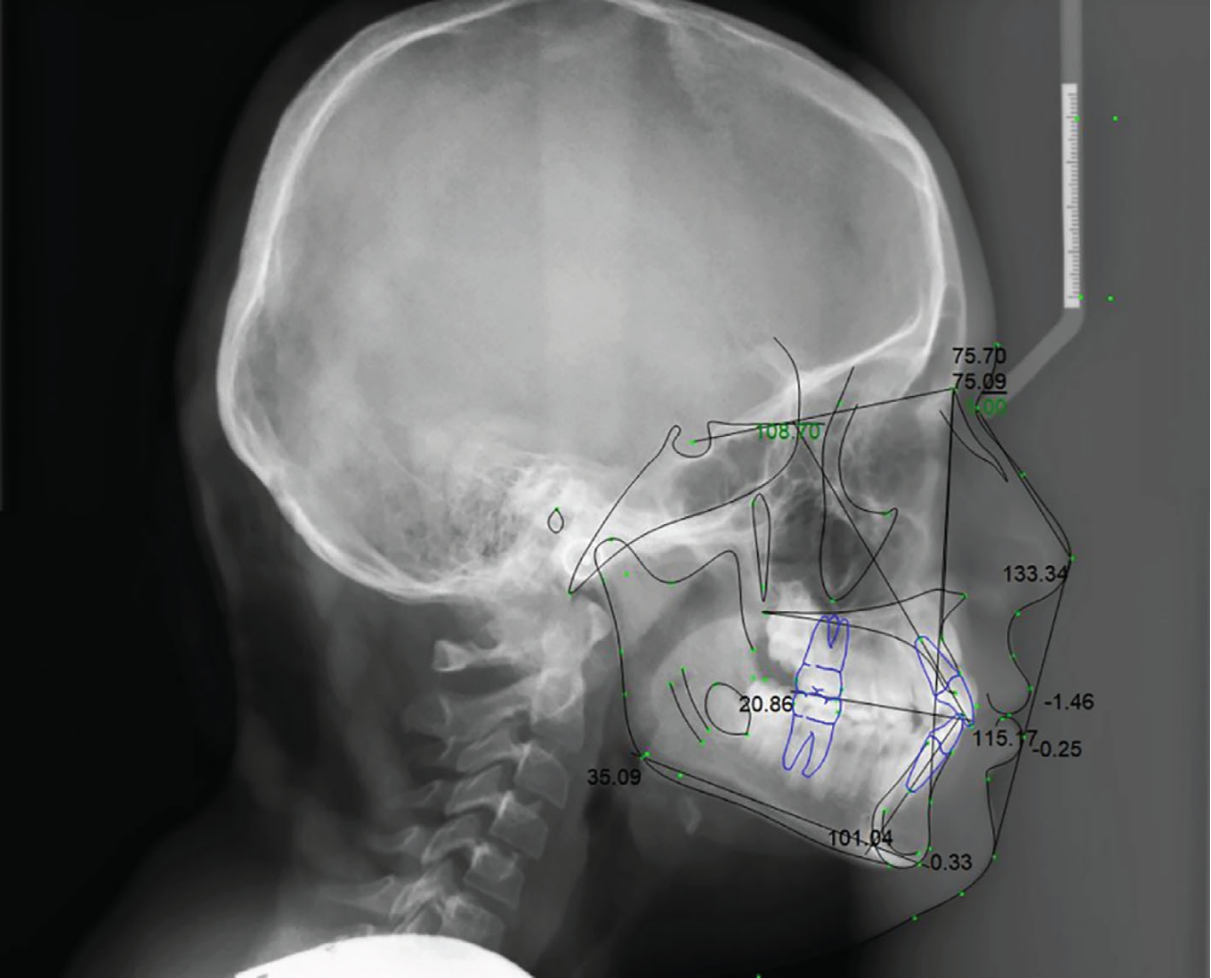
Pretreatment digitized lateral cephalometric radiograph.
